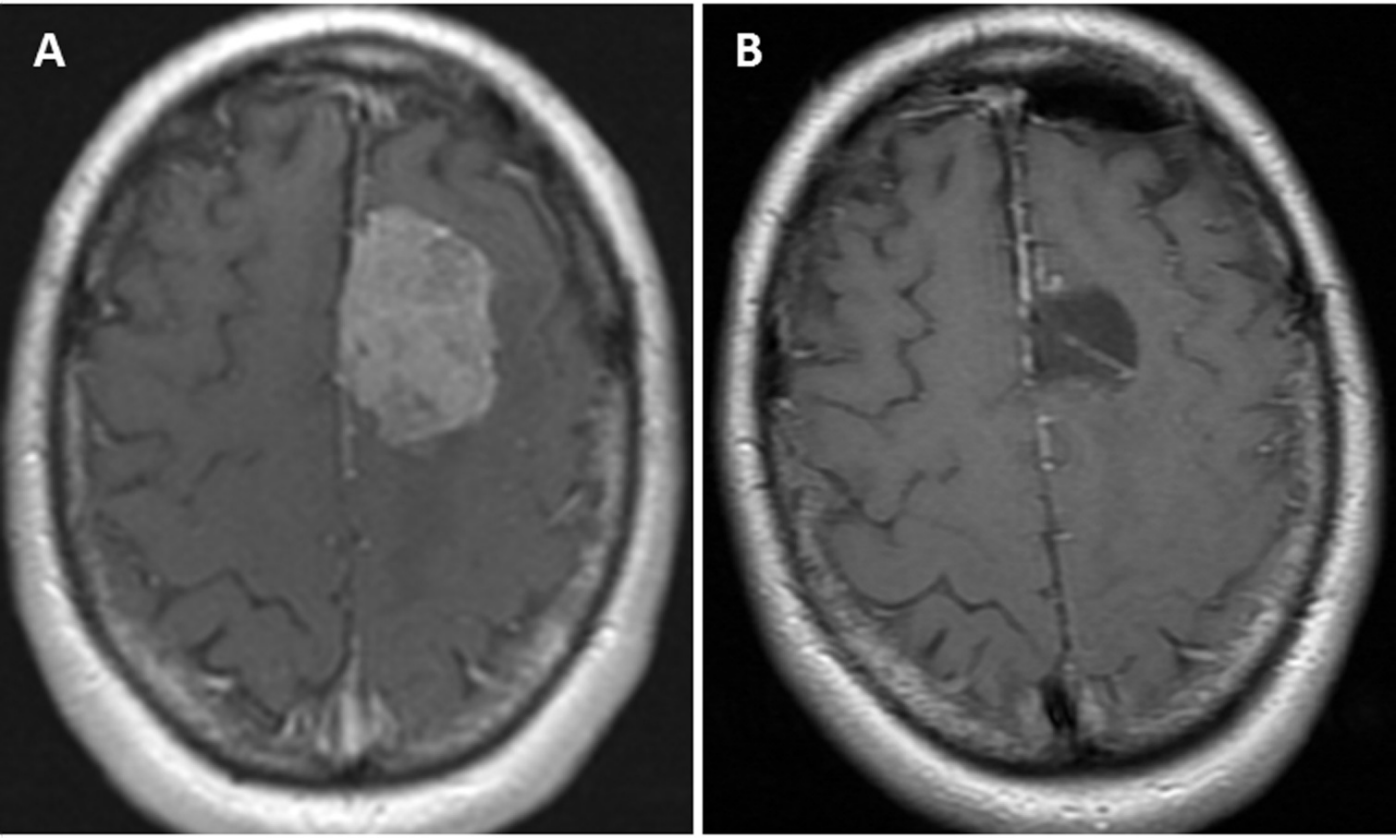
Tailored Keyhole Approach Preoperative post-contrast axial T1 MRI shows left falcine meningioma (A). Postoperative axial MRI shows complete resection (B).
